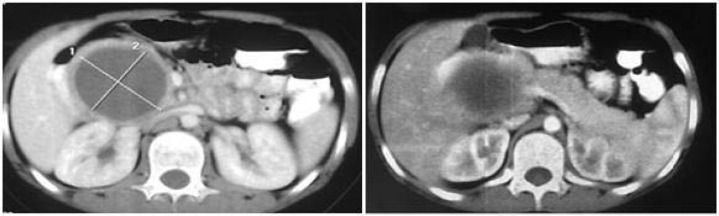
Axial view of abdomenal CT scan with a retroperitoneal mass with measuring 60*58 mm in size compressed liver and right kidney vessels
